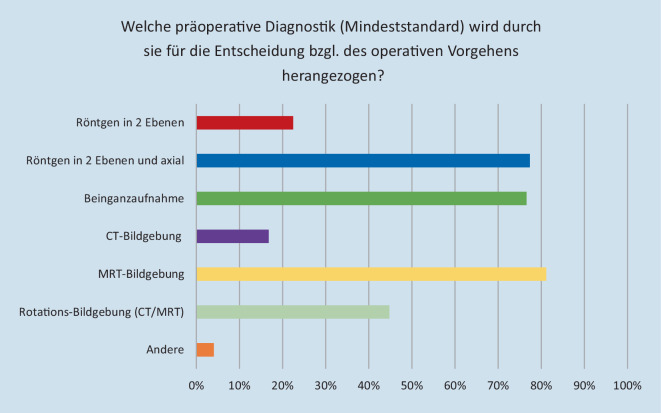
In der präoperativen nativradiologischen Diagnostik (Mehrfachnennung möglich) werden von den Teilnehmern der Umfrage Beinachse (91,6 %), Trochleaform (83,5 %), Patellahöhe (80,4 %), Patellaform (71,5 %) und Patella-Tilt (68,9 %) erhoben. Im Fall einer präoperativ durchgeführten CT-Diagnostik wird durch die Teilnehmer am Häufigsten der TTTG („tibial tuberosity – trochlear groove“)/TTPCL („tibial tuberosity – posterior cruciate ligament“) – Abstand erhoben (75,8 %), weitere erhobene Parameter sind: Trochleaform (68,5 %), Torsion (59,0 %), Patellaform (53,7 %) und Patellahöhe (45,0 %). Die für die präoperative Planung relevanten Parameter in der MRT-Bildgebung waren Knorpelverhältnisse (92,4 %), mediales Retinakulum/MPFL (88,6 %), Trochleaform (75,6 %), TTTG/TTPCL (72,8 %), Patellaform (57,8 %), Patellahöhe (47,3 %), Patella-Tilt (46,8 %) und Torsion (36,1 %).
